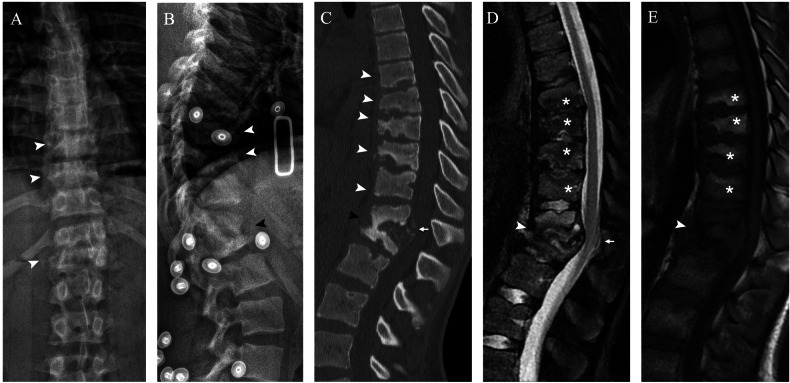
Neuropathic arthropathy of the thoracolumbar spine in a 15-year-old boy with hereditary sensory and autonomic neuropathy type IV, who presented intermittent claudication. Anteroposterior (A) and lateral (B) radiographs of the dorso-lumbar spine showing lytic changes of multiple vertebrae (white arrowhead) and collapse of T12-L1 (black arrowhead). Sagittal CT (C) confirms endplate destruction from T7 to T11 (white arrowhead), L1 collapse with partial fusion of T12-L1 (black arrowhead), and intracanal bone fragments (arrow). Sagittal T2 (D) and T1 (E) MRI confirm the intracanal protrusion of the posterior wall of L1 (arrow), fragmentation and oedema of T12-L1 (white arrowhead) as well as endplate lytic changes and degenerative fatty changes of bone marrow in multiple thoracic vertebrae (asterisk)
